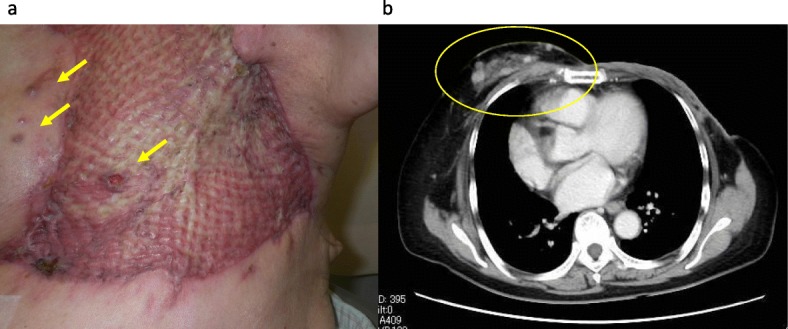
The following two recurrences appeared 40 days after surgery: a Local recurrence on the skin graft and skin metastases of the contralateral breast (indicated by arrows) and b Enhanced nodules in the contralateral breast as detected by MRI (circled)
